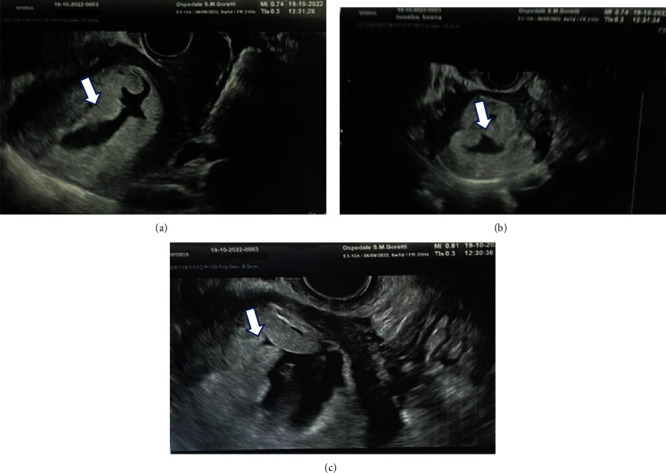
Pelvic TV ultrasound at 6 weeks of amenorrhea in longitudinal (a), transverse (b), and magnified scan (c). Ultrasound scan show a small embrionary site without cardiac activity and a diffuse trophoblast with an irregular and breasted profile in its intracavitary portion. The pictures depose for internal abortion and suspected molar degeneration.
